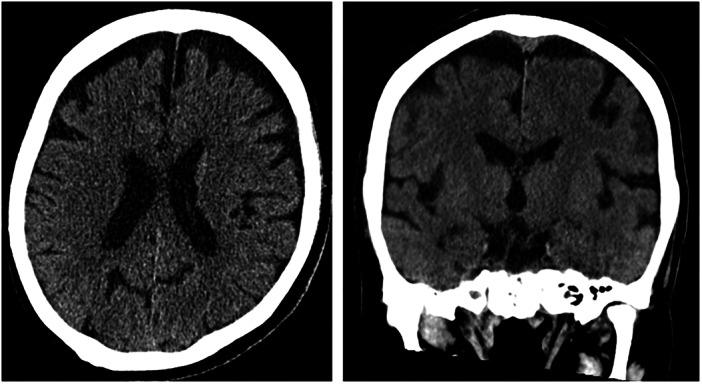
Computed tomography of the head on the 29th day in the hospital showing preserved hippocampal volume but significant atrophy of the frontal lobe.
